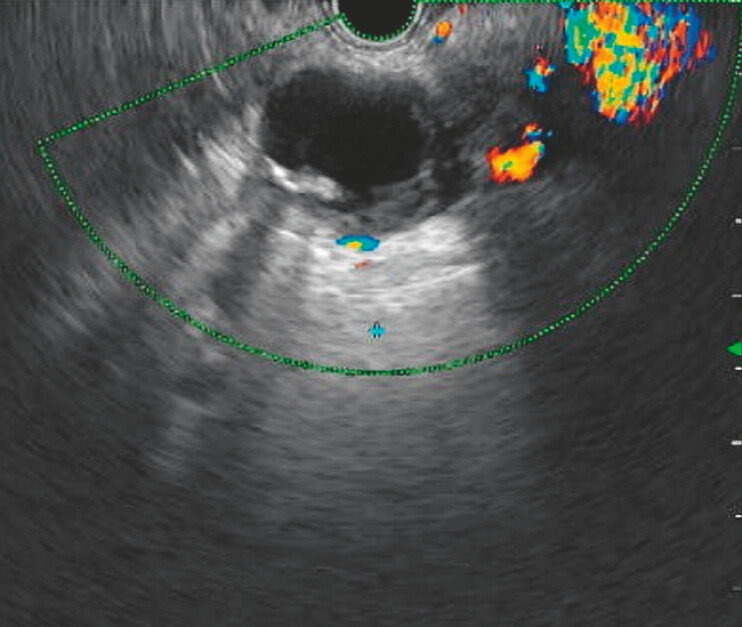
EUS images reveal dilation of the main PD in the tail of the pancreas, along with a fluid-attenuated area in the pancreatic body. EUS, endoscopic ultrasound; PD, pancreatic duct drainage.
